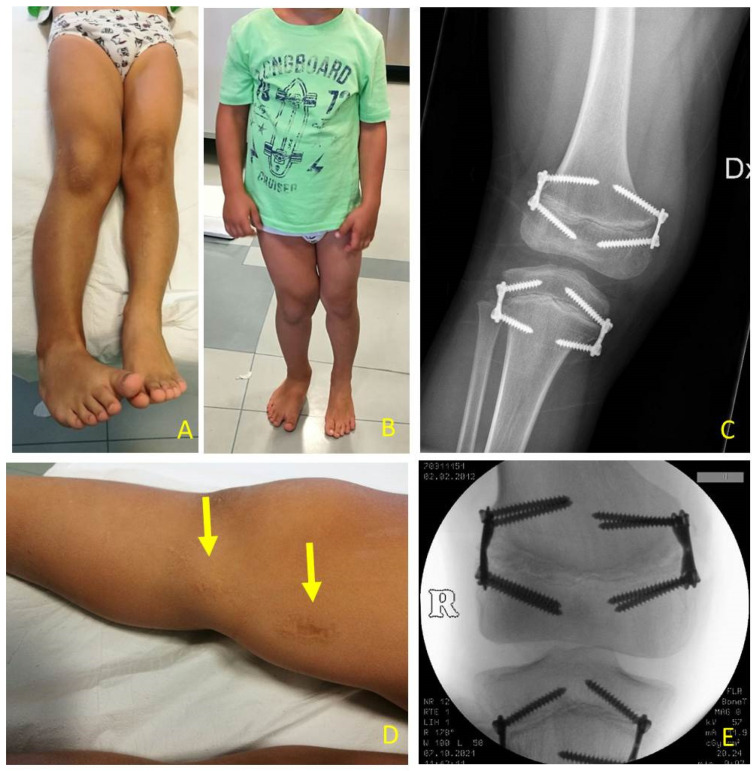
Same patient as shown in Figure 7, at age 6.7 years: (A) LLD (leg length discrepancy) decreased but valgus deformity is developing; (B) clinical aspect in standing position; (C) X-ray of right knee shows valgus deformity and divergent aspects of screws; (D) small skin scars after first surgical procedures (yellow arrows); (E) X-ray on knee in antero-posterior projection shows on femur substitution of eight-plate with stronger Quad-plates to avoid increasing valgus deformity (8-plateR and quad-plateR; Eight Plate Guided Growth System+, Orthofix Medical Inc.© Lewisvill, TX (USA)).
